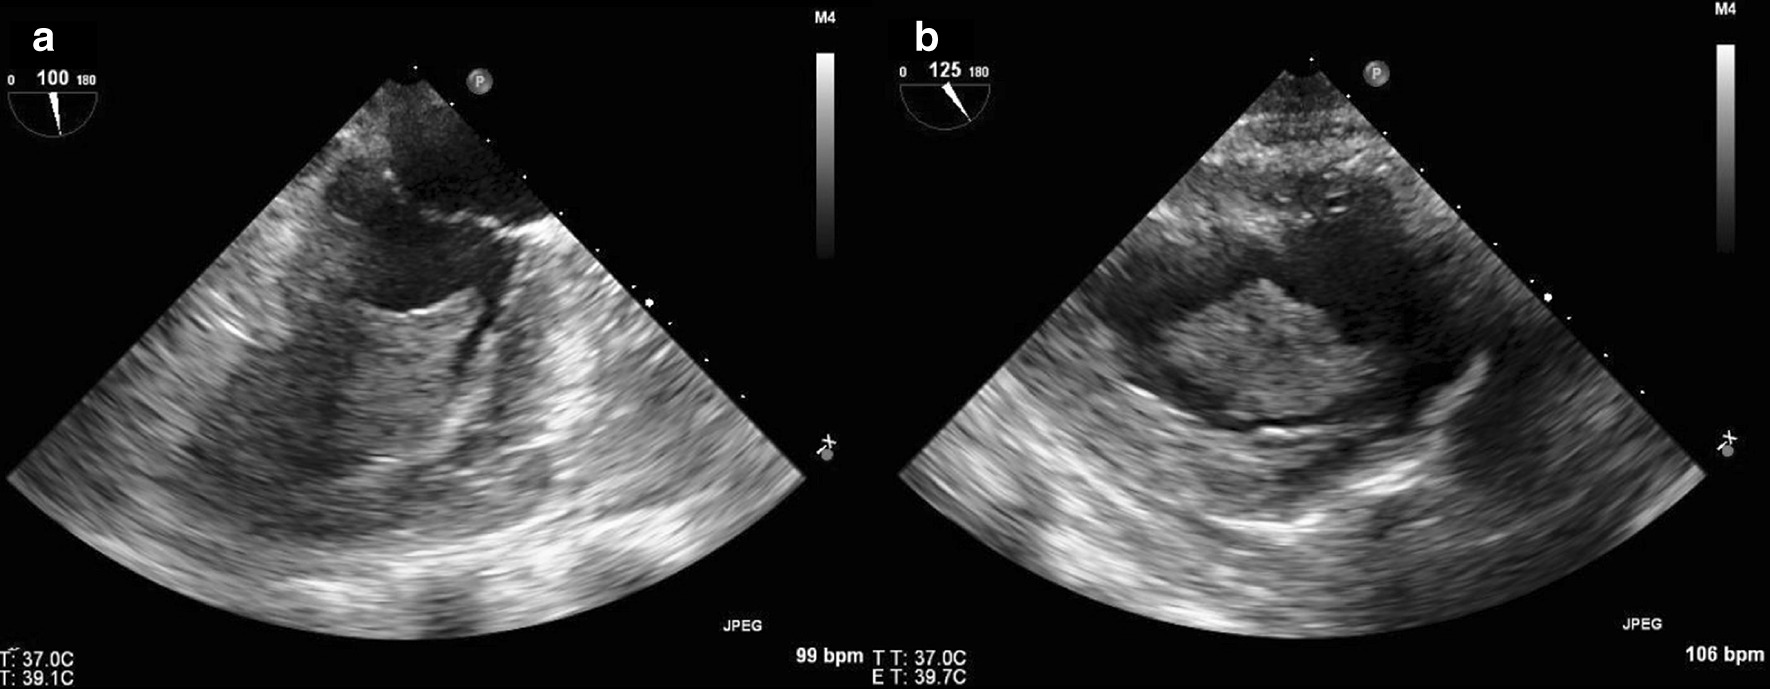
Case 3. Transesophageal echocardiographic (TEE) mid-esophageal 2 chamber and transgastric projections
